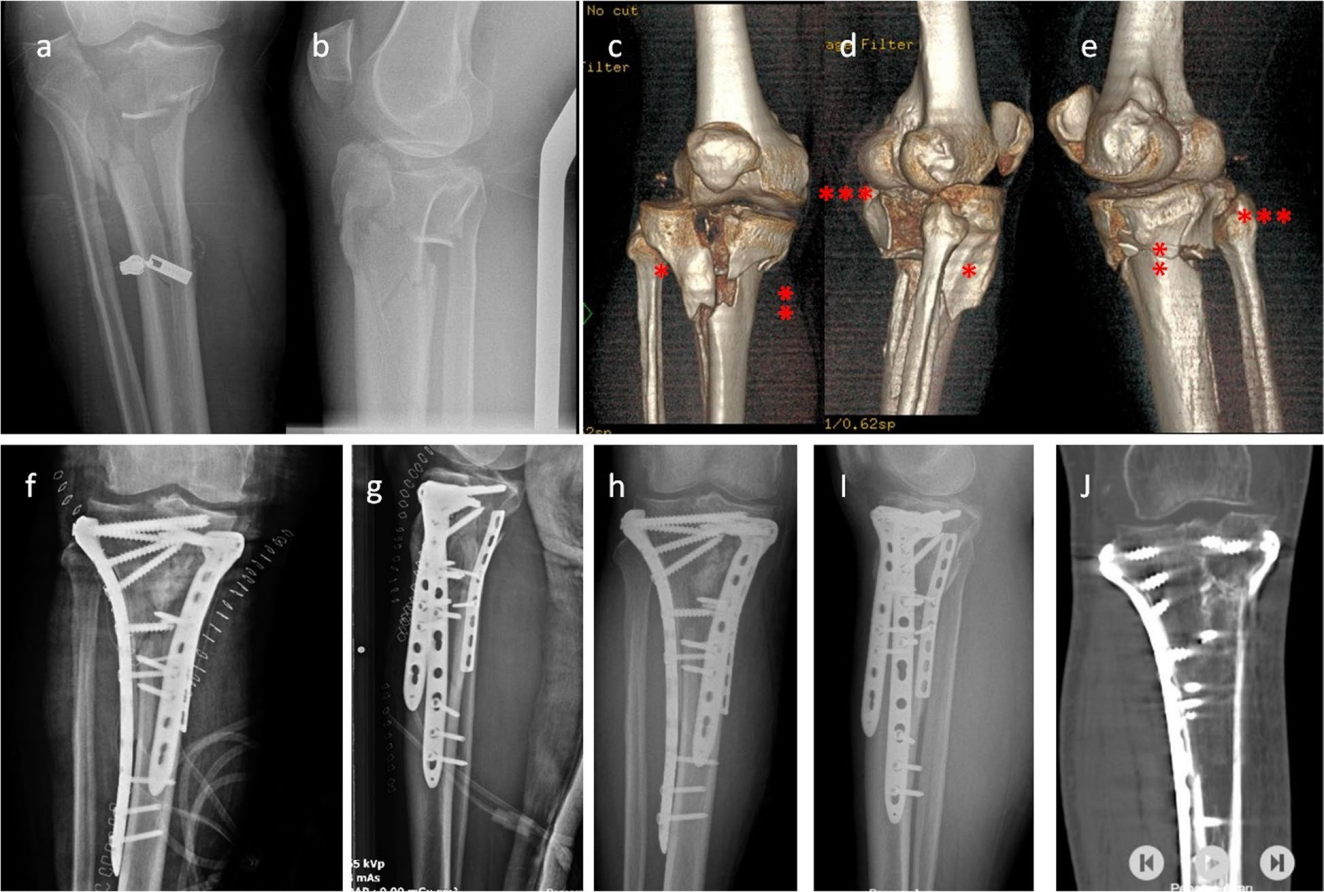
a, b A patient sustaining right tibia plateau fracture after a motor vehicle accident. c–e 3D CT revealed fractures of three columns. *Lateral column, medial column, *posterior column. f, g The patient was treated using two incisions for fixation of three columns with three plates. Acceptable reduction was obtained during surgery. h–j X-ray and CT at 13 months postoperatively showed perfect union of the fracture
